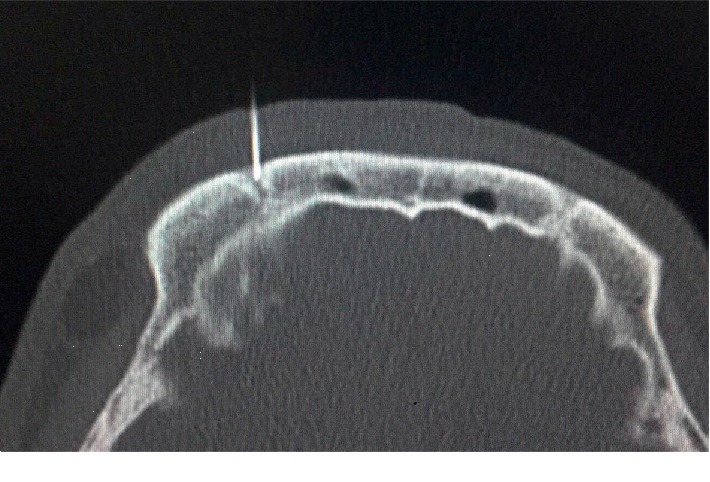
Insertion of puncture needle into the supraorbital foramen under the guidance of CT.
